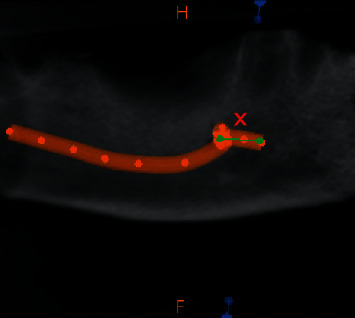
CBCT image showing anterior extension of alveolar nerve loop.
